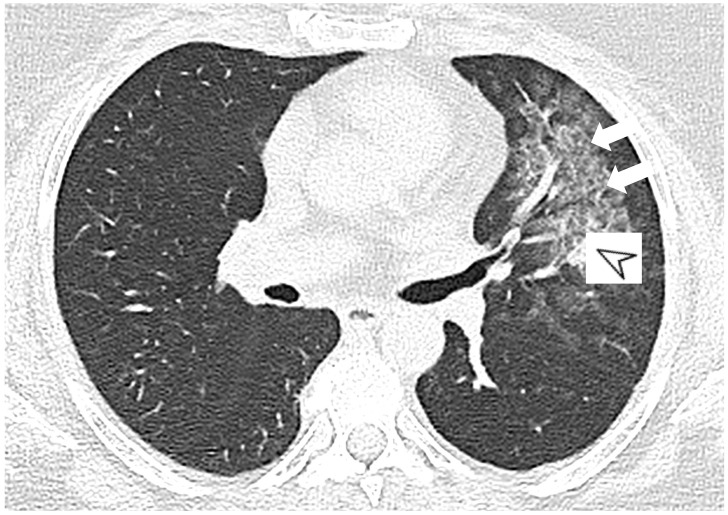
A 52-year-old female with MPA vasculitis. Axial high-resolution CT shows GGO in the left upper lobe (white arrows), due to alverolar hemorrhage; a peribronchial opacity is also depicted in the pulmonary parenchyma (white arrowhead).
